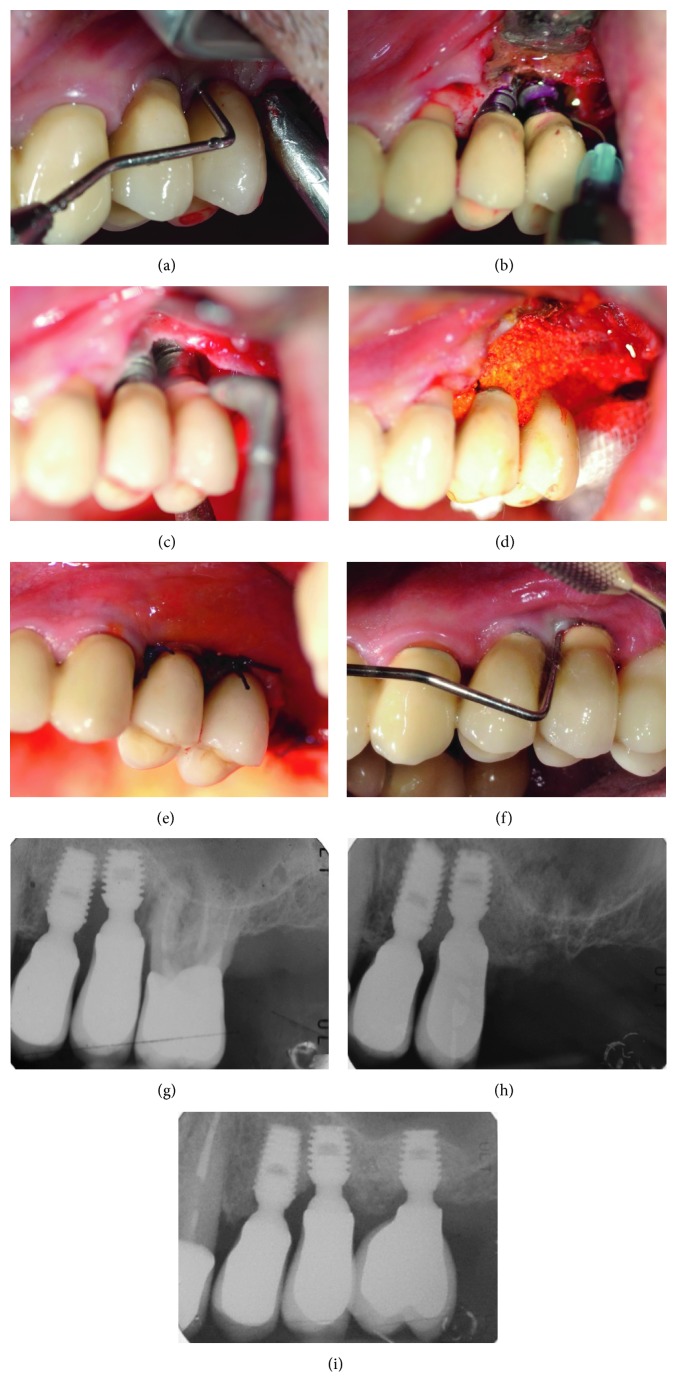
Regenerative treatment of peri-implantitis in the upper posterior jaw in a male patient. (a) Clinical probing before treatment. (b) Application of desiccant. (c) Decontamination with low abrasive air powder. (d) Filling of the peri-implant bone defect. (e) Postoperative clinical aspect. (f) Clinical follow-up 5 years after surgical treatment. (g) Preoperative X-ray. (h) Postoperative X-ray. (i) 5-year follow-up.
